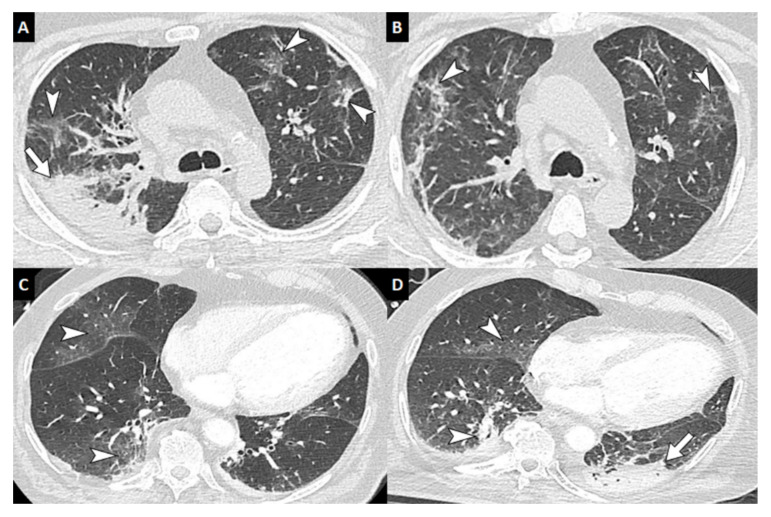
Computed tomography of the chest of patients with COVID-19 with secondary invasive aspergillosis. Unenhanced chest CT in a 59-year-old man with COVID-19 and biological markers of invasive aspergillosis performed at baseline (A) and at 12-day follow-up (B) showing subpleural ground-glass and reticular opacities presumed to correspond to COVID-19 lesions (arrowheads) as well as a right apical consolidation area presumed to correspond to invasive aspergillosis (arrow). Enhanced chest CT in a 69-year-old man with COVID-19 and biological markers of invasive aspergillosis showing at baseline (C) ground-glass opacities (arrowheads), and at 11-day follow-up (D) a left postero-basal consolidation presumed to correspond to invasive aspergillosis (arrow). (346-mm field of view, 512 × 512 image matrix, lung window (W1600/L-500 HU)).
